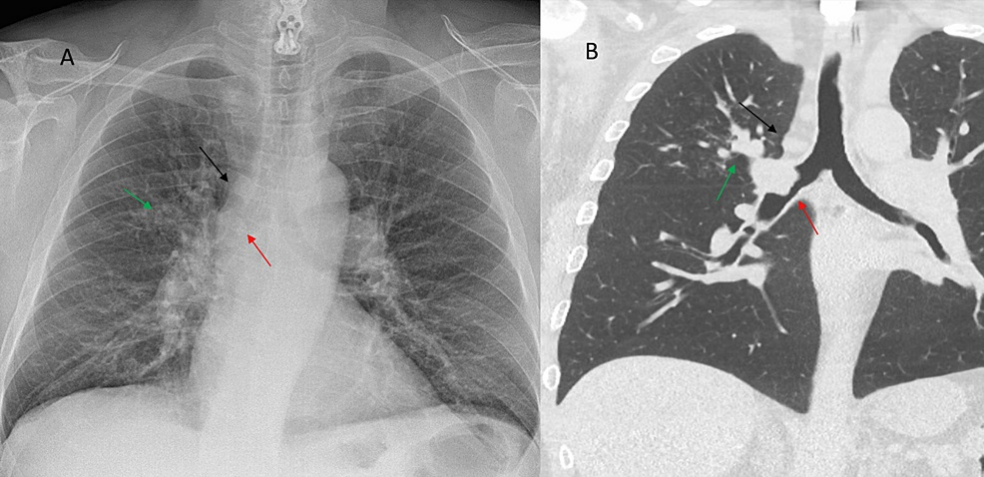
Last chest X-ray realized (A) and corresponding CT section (B). The red arrow shows the endobronchial mass. The black arrow shows the widening of the right paratracheal stripe and the mediastinal adenomegaly. The green arrow shows the distal mucus plugging in the right upper lobe.
